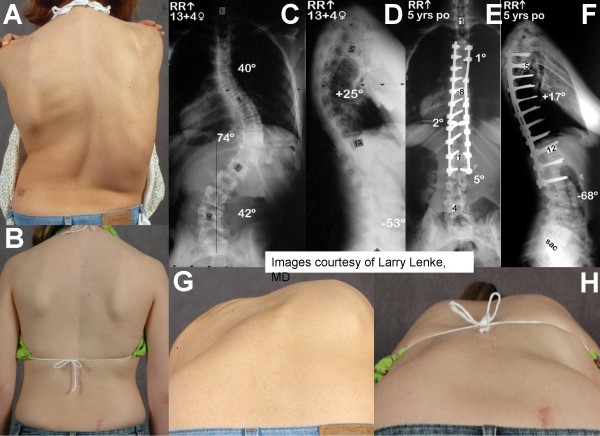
Case example of a 13 year-old old female with progressive AIS. Clinical photographs show spinal imbalance, and elevated right shoulder (preoperative panels A, G). Adam's forward bend test shows right thoracic prominence correlating with vertebral rotation and right rib elevation. Radiographs in postero-anterior and lateral views show a proximal thoracic curve of 40°, a main thoracic curve of 74°, and a lumbar curve of 42° (panel C), with normal sagittal parameters (panel D). A posterior correction with fusion from T4-L2 was performed. Five years postoperatively, radiographs show a 97% correction of the proximal and main thoracic curves with a good maintenance of sagittal balance (panels E, F). Clinical photographs at 5 years show a restoration of spinal balance (panel B). The Adam's forward bend shows reduction of thoracic rib prominence (panel H). The pictures were kindly provided by courtesy of Dr. Larry Lenke.
